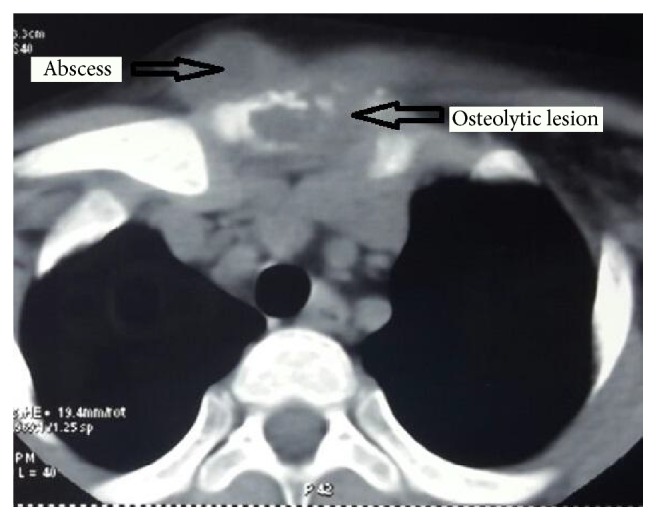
Computed tomography image demonstrating lytic destruction of manubrium with overlying peripherally enhancing soft tissue which is suggestive of osteomyelitis of the manubrium with abscess formation in the adjacent tissue.
